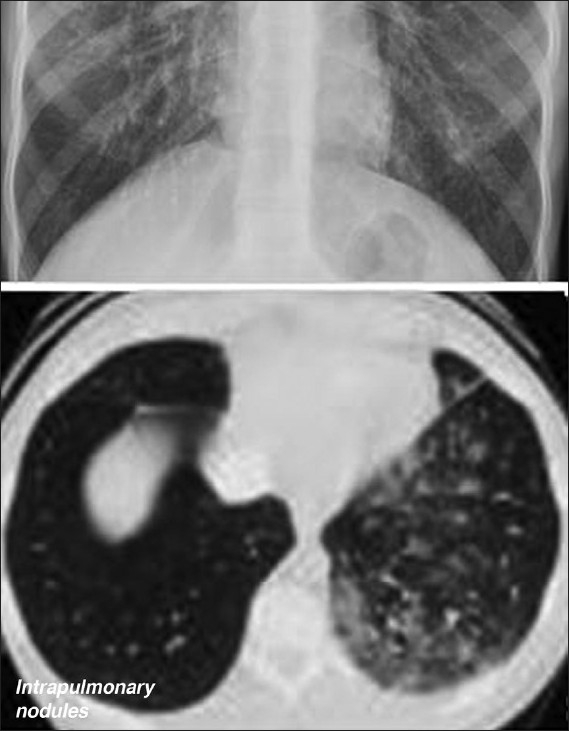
A coned view of a CXR of lung basis with HPS shows bibasilar nodular or reticulonodular opacities. These are seen in 5–13.8% of patients with CLD and 46–100% of patients with HPS. These opacities are shown to represent dilated lung vessels on computed tomography as shown
